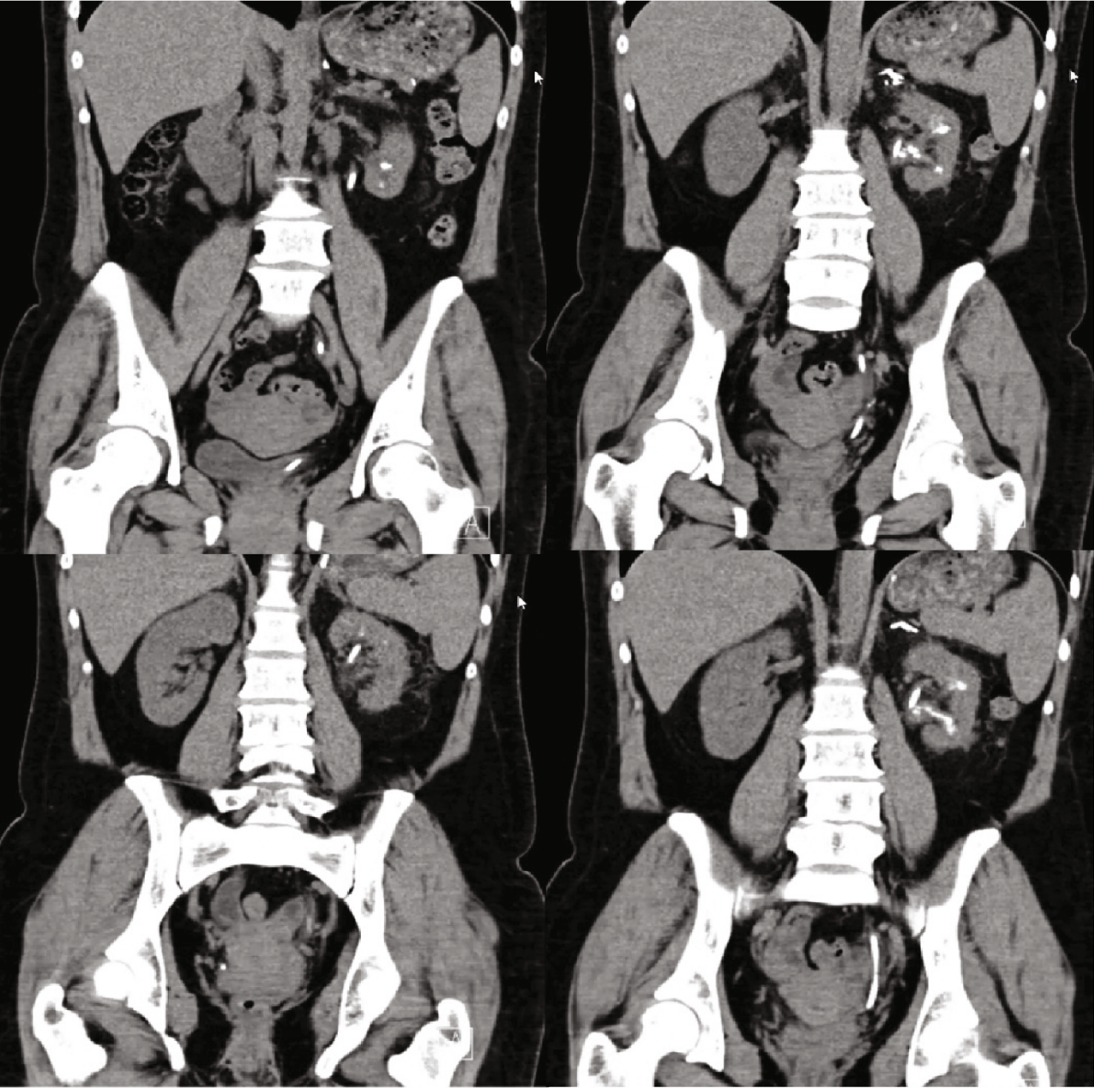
Case 1 abdominopelvic CT scan (after flexible RIRS with thulium laser). The CT scan shows residual lithiasis of small size (including intraparenchymal stones) and a double J stent.
